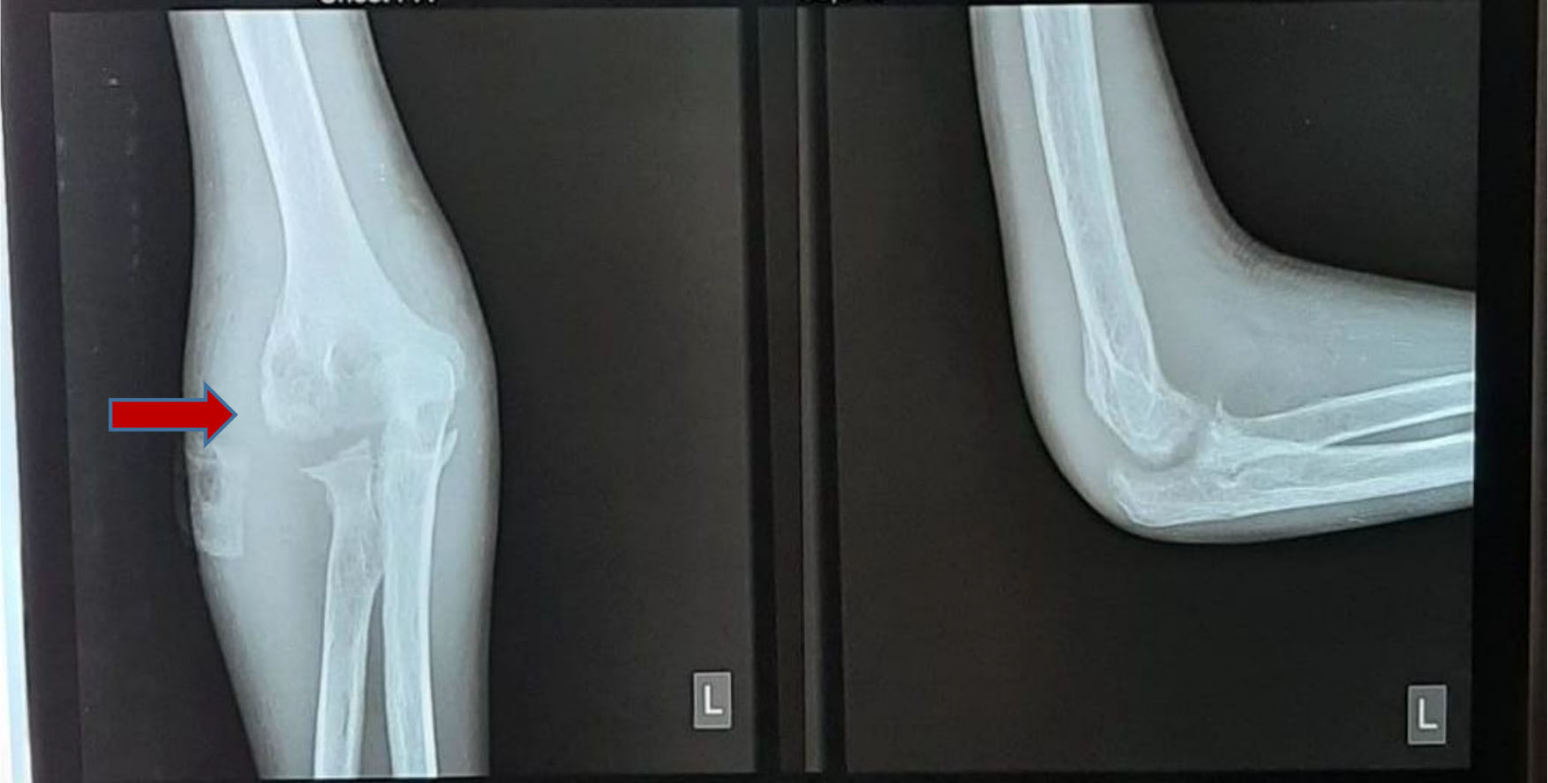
Anteroposterior and lateral projection of the left elbow joint radiographs showed erosion of the distal cortex of the humerus and radial bone, destruction of the distal cortex, and swelling of the soft tissue of the left elbow area (February 2021).
